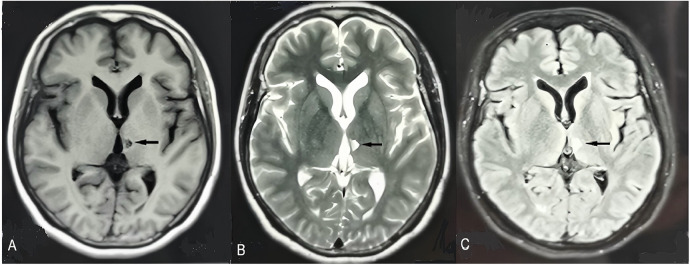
Brain magnetic resonance imaging obtained 3 months after hospital discharge. T1- weighted imaging shows hypointensity in the left thalamus (A), with a small corresponding hyperintense lesion on T2-weighted and fluid-attenuated inversion recovery images [(B) and (C), black arrow].
